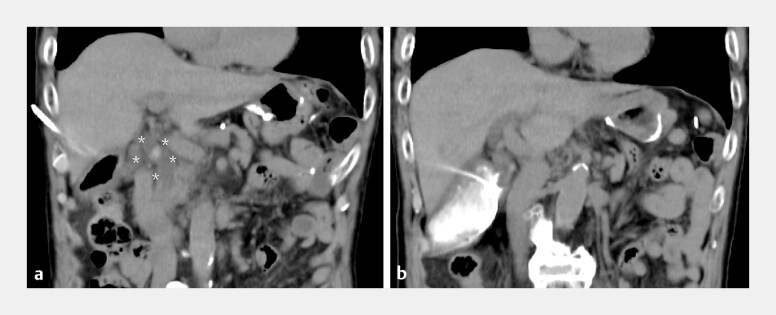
Abdominal computed tomography (CT) images.
